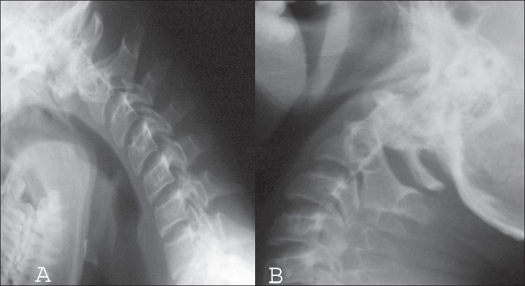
Lateral X-rays of cervical spine (flexion - extension) shows a reducible AAD (3A-Flexion, 3B Extension)
